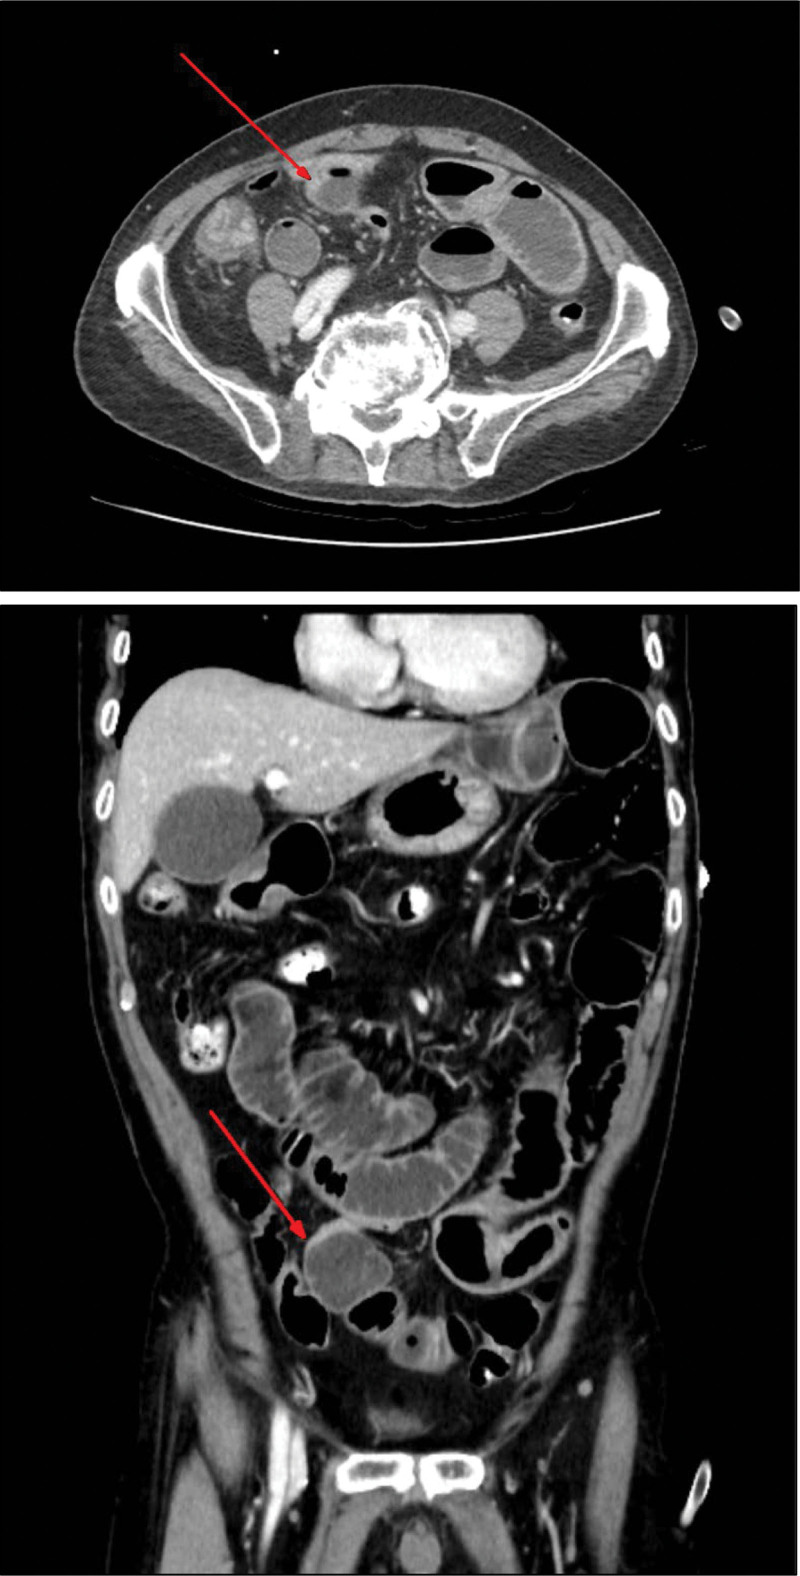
Initial CT abdomen and pelvis. Dilatation of small bowel with abrupt narrowing (red arrow) at the distal ileum suggests simple mechanical obstruction due to the adhesion band.
